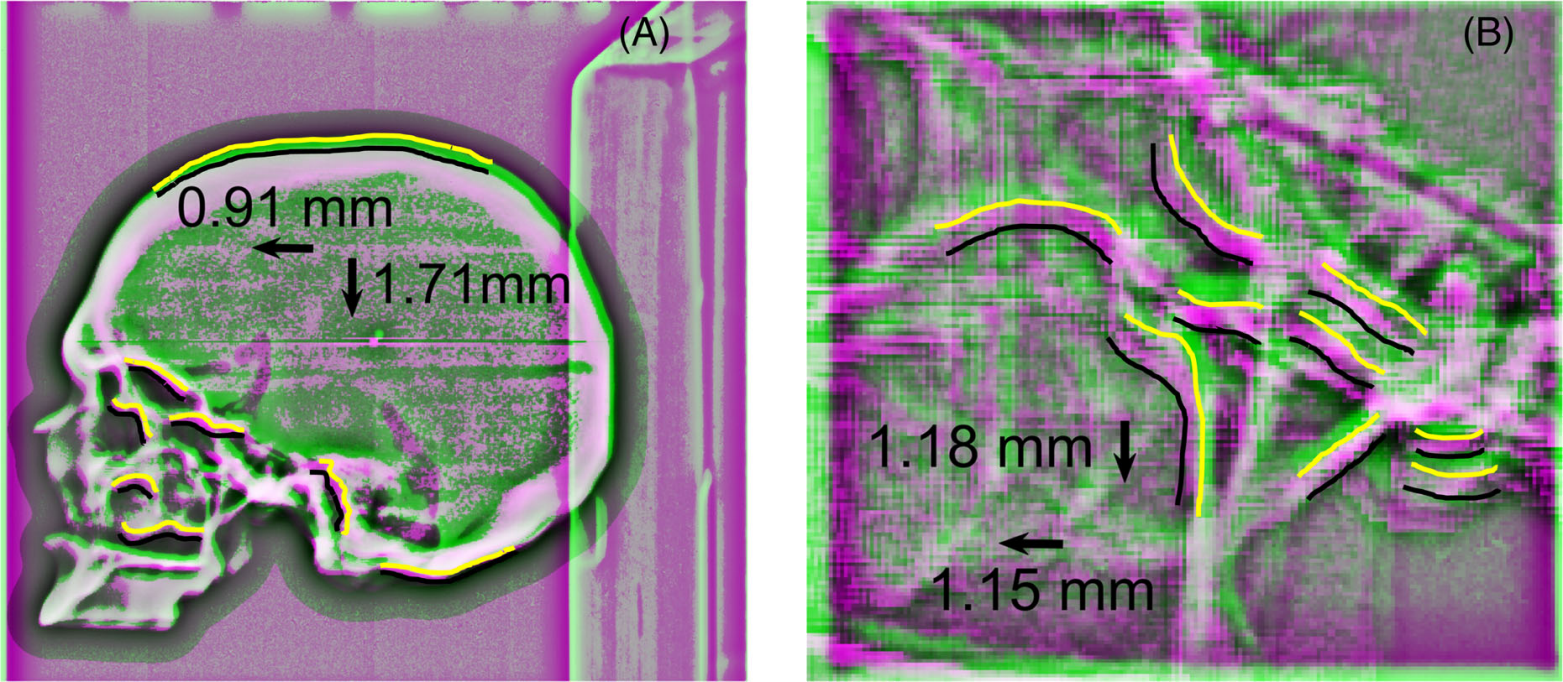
Example of anatomical registration between megavoltage (MV) image with the electronic portal imaging device (EPID) (green overlay) and a MATLAB‐generated digitally reconstructed radiograph (DRR) (magenta overlay). Yellow lines were drawn to highlight example edges of anatomical features in the EPID image, whereas black lines depict the same example edges in the DRR: (a) registration with an open field (22 × 22 cm^2^); (b) registration with an 80% thresholded aperture described in Figure 3. The detected shifts for this control point are shown for the case of a sudden 1.5 mm shift along each linear couch axis with the gantry at 90 degrees and the couch at 0 degrees.
